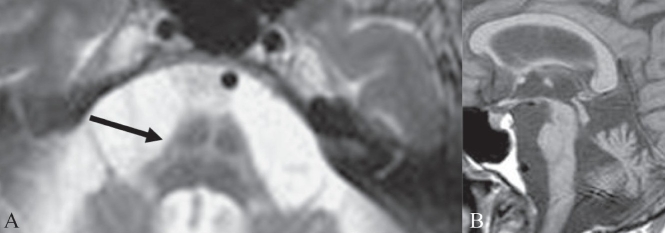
Hot cross bun sign. Axial T2W MRI image of the pons (A) in a patient with multiple system atrophy shows a hyperintense linear area forming a ‘cross’ (arrow) in the pons. Sagittal T1W image (B) shows atrophy of the cerebellum and cerebrum (prominence of sulci)
